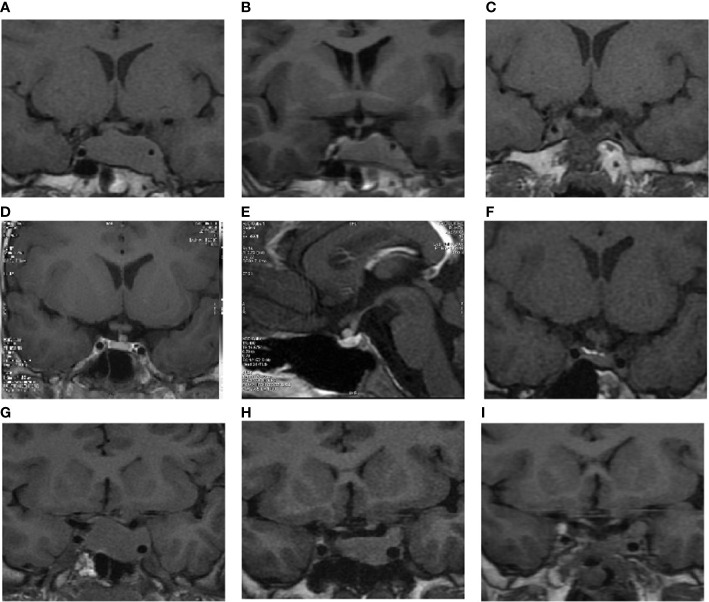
The pituitary MRI imaging of cases 1, 2, and 3. (A) Coronal imaging of case 1 during the first visit. (B) Coronal imaging of case 1 after long-term SSA therapy. (C) Coronal imaging of case 1 during the last follow-up. (D) An ectopic pituitary stalk tumor in case 2 during the first visit. (E) Sagittal pituitary imaging of case 2 during the first visit. (F) Coronal pituitary imaging of case 2 during the last follow-up. (G) Coronal imaging of case 3 during the first visit. (H) Coronal imaging of case 3 after long-term SSA therapy. (I) Coronal imaging of case 3 at the last follow-up.
